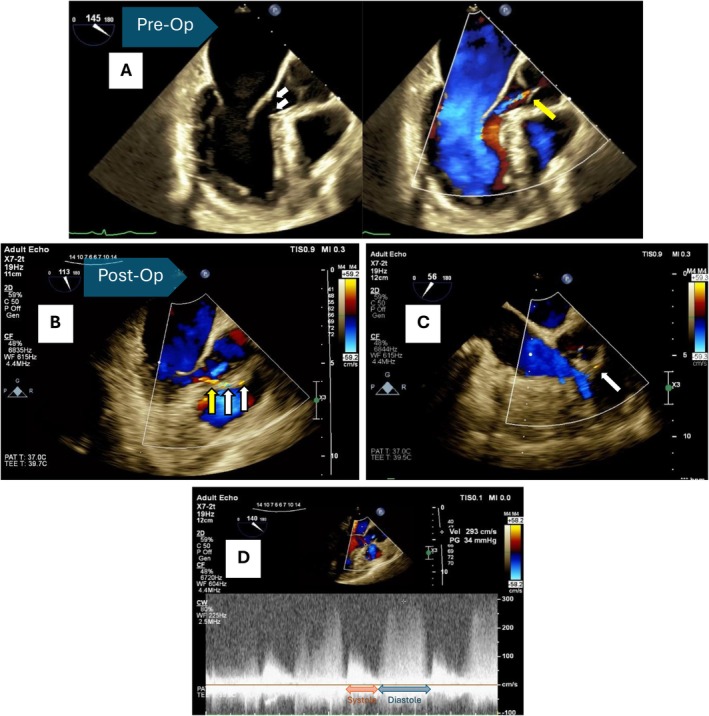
Transesophageal echocardiography; pre‐operative (A) and post‐operative (B–D). (A) *Preoperative* aortic long‐axis views without (left) and with (right) color Doppler showing a subaortic web (white arrows). The only diastolic flow is aortic regurgitation (yellow arrow), with no abnormal fistula flow. (B) *Postoperative* aortic long‐axis view with color Doppler demonstrating an abnormal diastolic flow from the basal interventricular septum into the left ventricular outflow tract (LVOT) (white arrow), with oblique orientation of the exit jet directed away from the interventricular septum (yellow arrow), consistent with coronary–cameral fistula. (C) *Postoperative* aortic short‐axis view with color Doppler showing a cross‐sectional image of the same fistulous tract arising from the basal interventricular septum in diastole (white arrow). (D) Continuous‐wave Doppler aligned with the jet, revealing diastolic flow (2.93 m/s).
